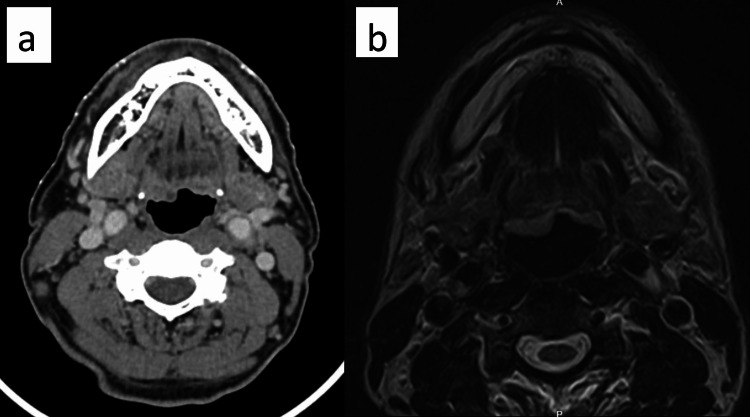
a: The CT scan showed thickening at the right palatine tonsil, retromolar trigone, and anterior and posterior tonsillar pillars. b: MRI showed an ill-defined lesion at the right retromolar trigone, laterally involving the right buccinator muscle.
